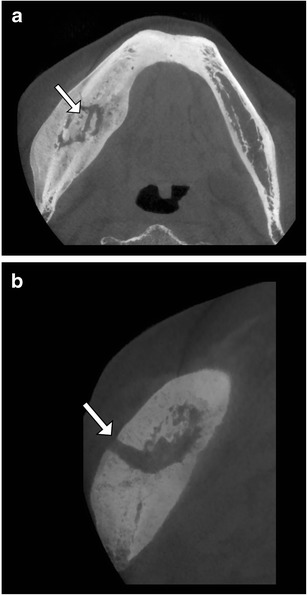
Chronic osteomyelitis of the right hemimandible. a. Axial CBCT reformatted image showing chronic osteomyelitis of the right hemimandible with intralesional sequestrum (arrow). Note marked sclerosis of the right hemimandible compared to the left side and massive periosteal bone reaction (involucrum). b. Detailed axial view shows the course of a fistula through the mandibular cortex to the buccal soft tissues (arrow)
